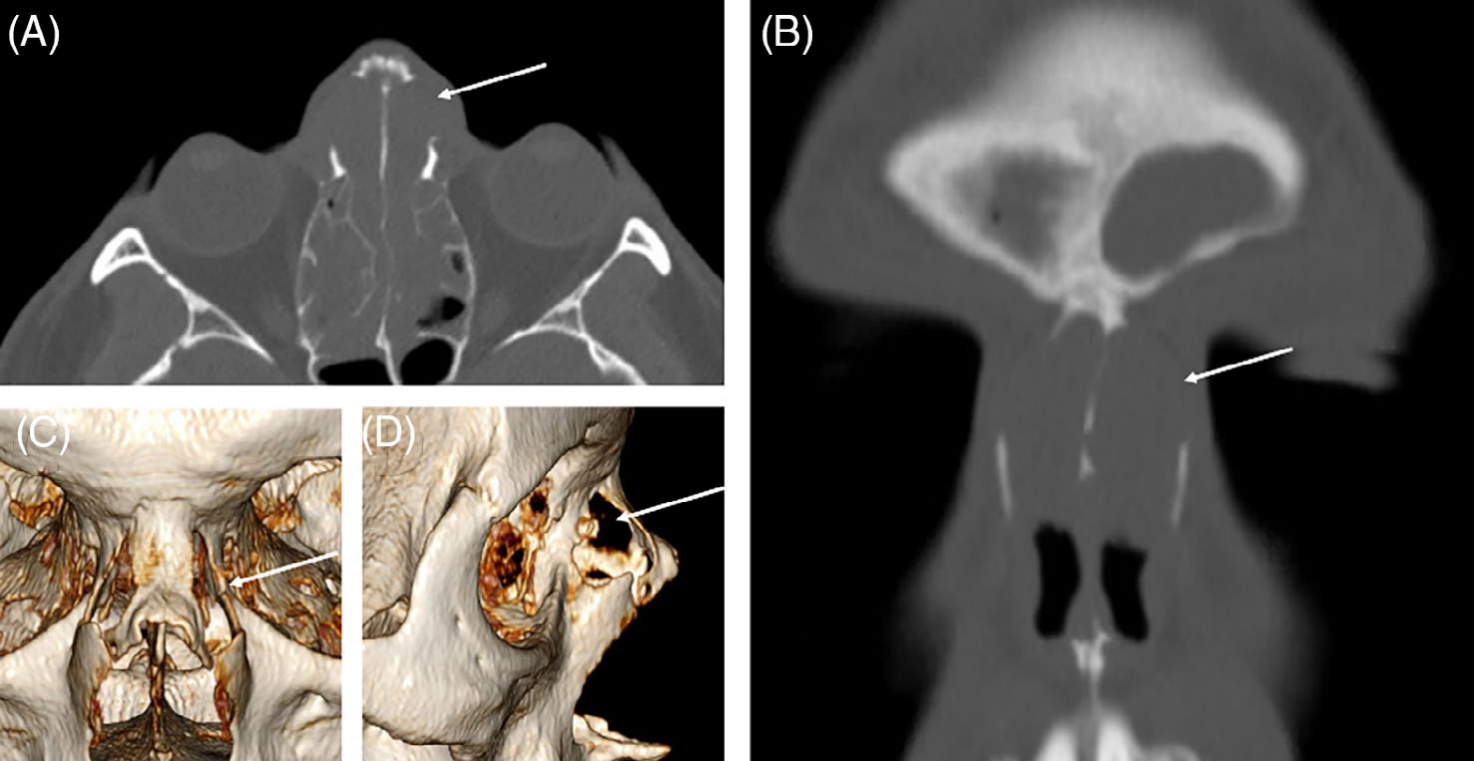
Case One: Preoperative axial (A) and coronal (B) CT planes demonstrating near‐complete rarefication of the lateral nasal bones (white arrow). Three‐dimensional reformats in frontal (C) and lateral (D) projections elucidate the bony deficit
